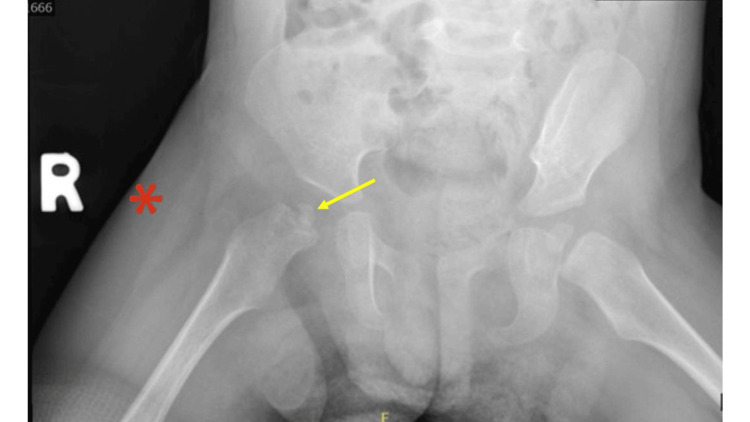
Anteroposterior radiograph of the pelvis. There is evidence of right (red asterisk) femoral head destruction, marked with yellow arrow, consistent with advanced joint damage following septic arthritis
